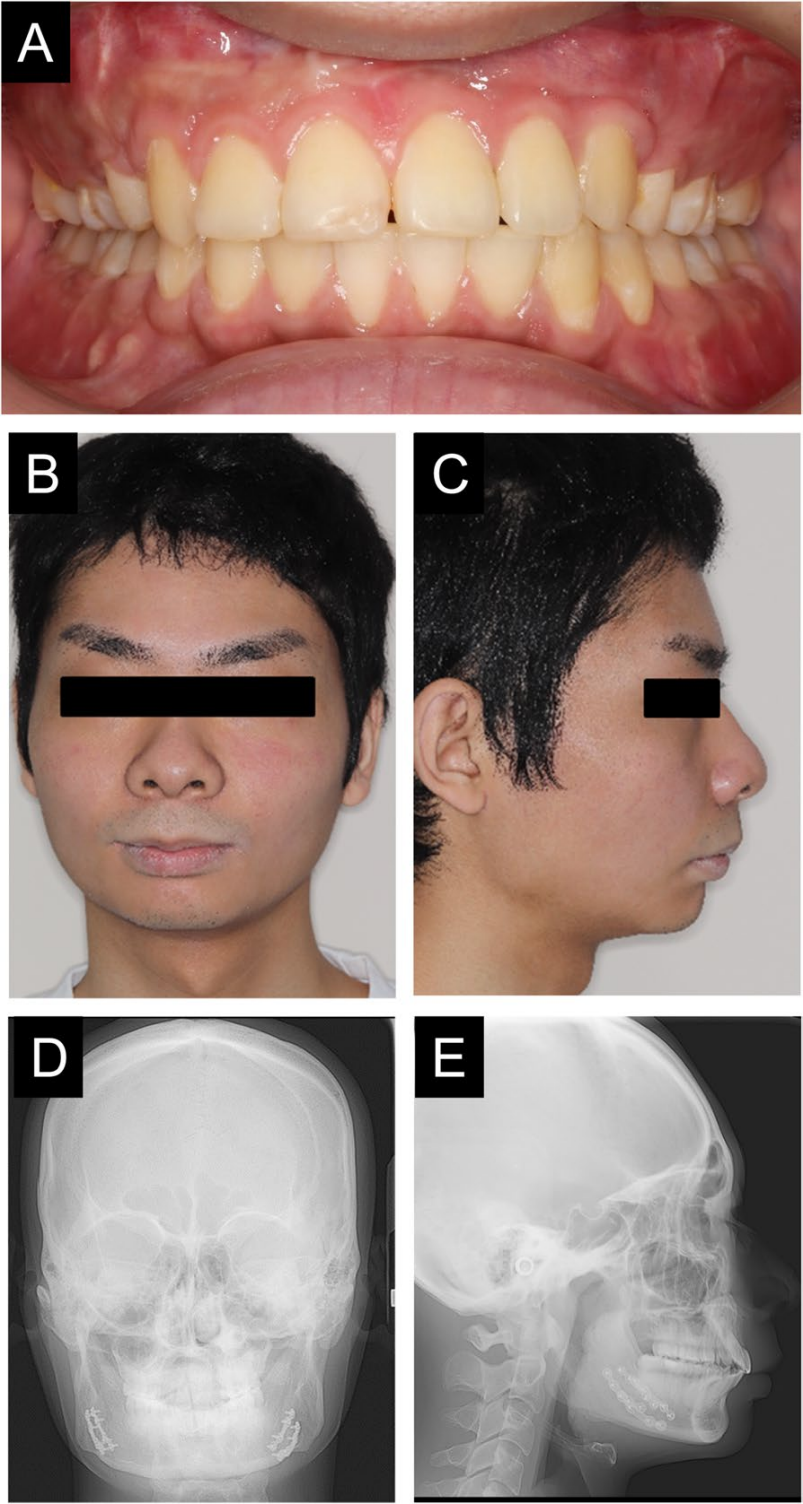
Postoperative photographs and radiographs. Intraoral photographs after orthognathic surgery (A). Frontal view (B) and lateral view (C) on facial photographs after orthognathic surgery. Frontal cephalogram (D) and lateral cephalogram (E) showing radiographic findings after orthognathic surgery
